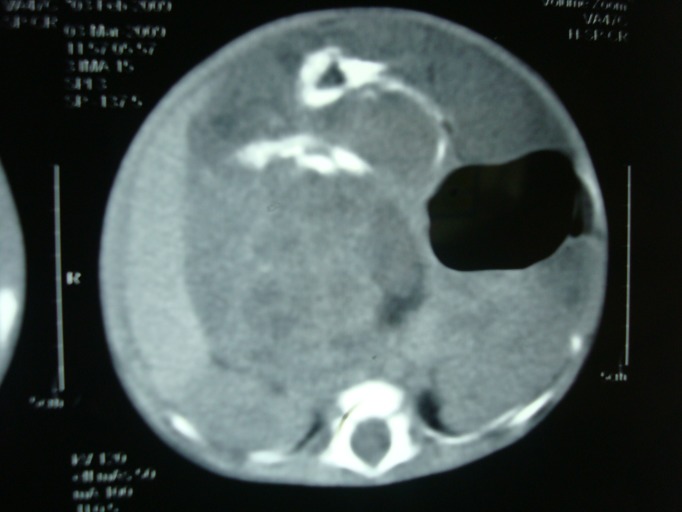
Figure 1: CT scan showing a mass with solid and cystic components along with internal calcifications.
